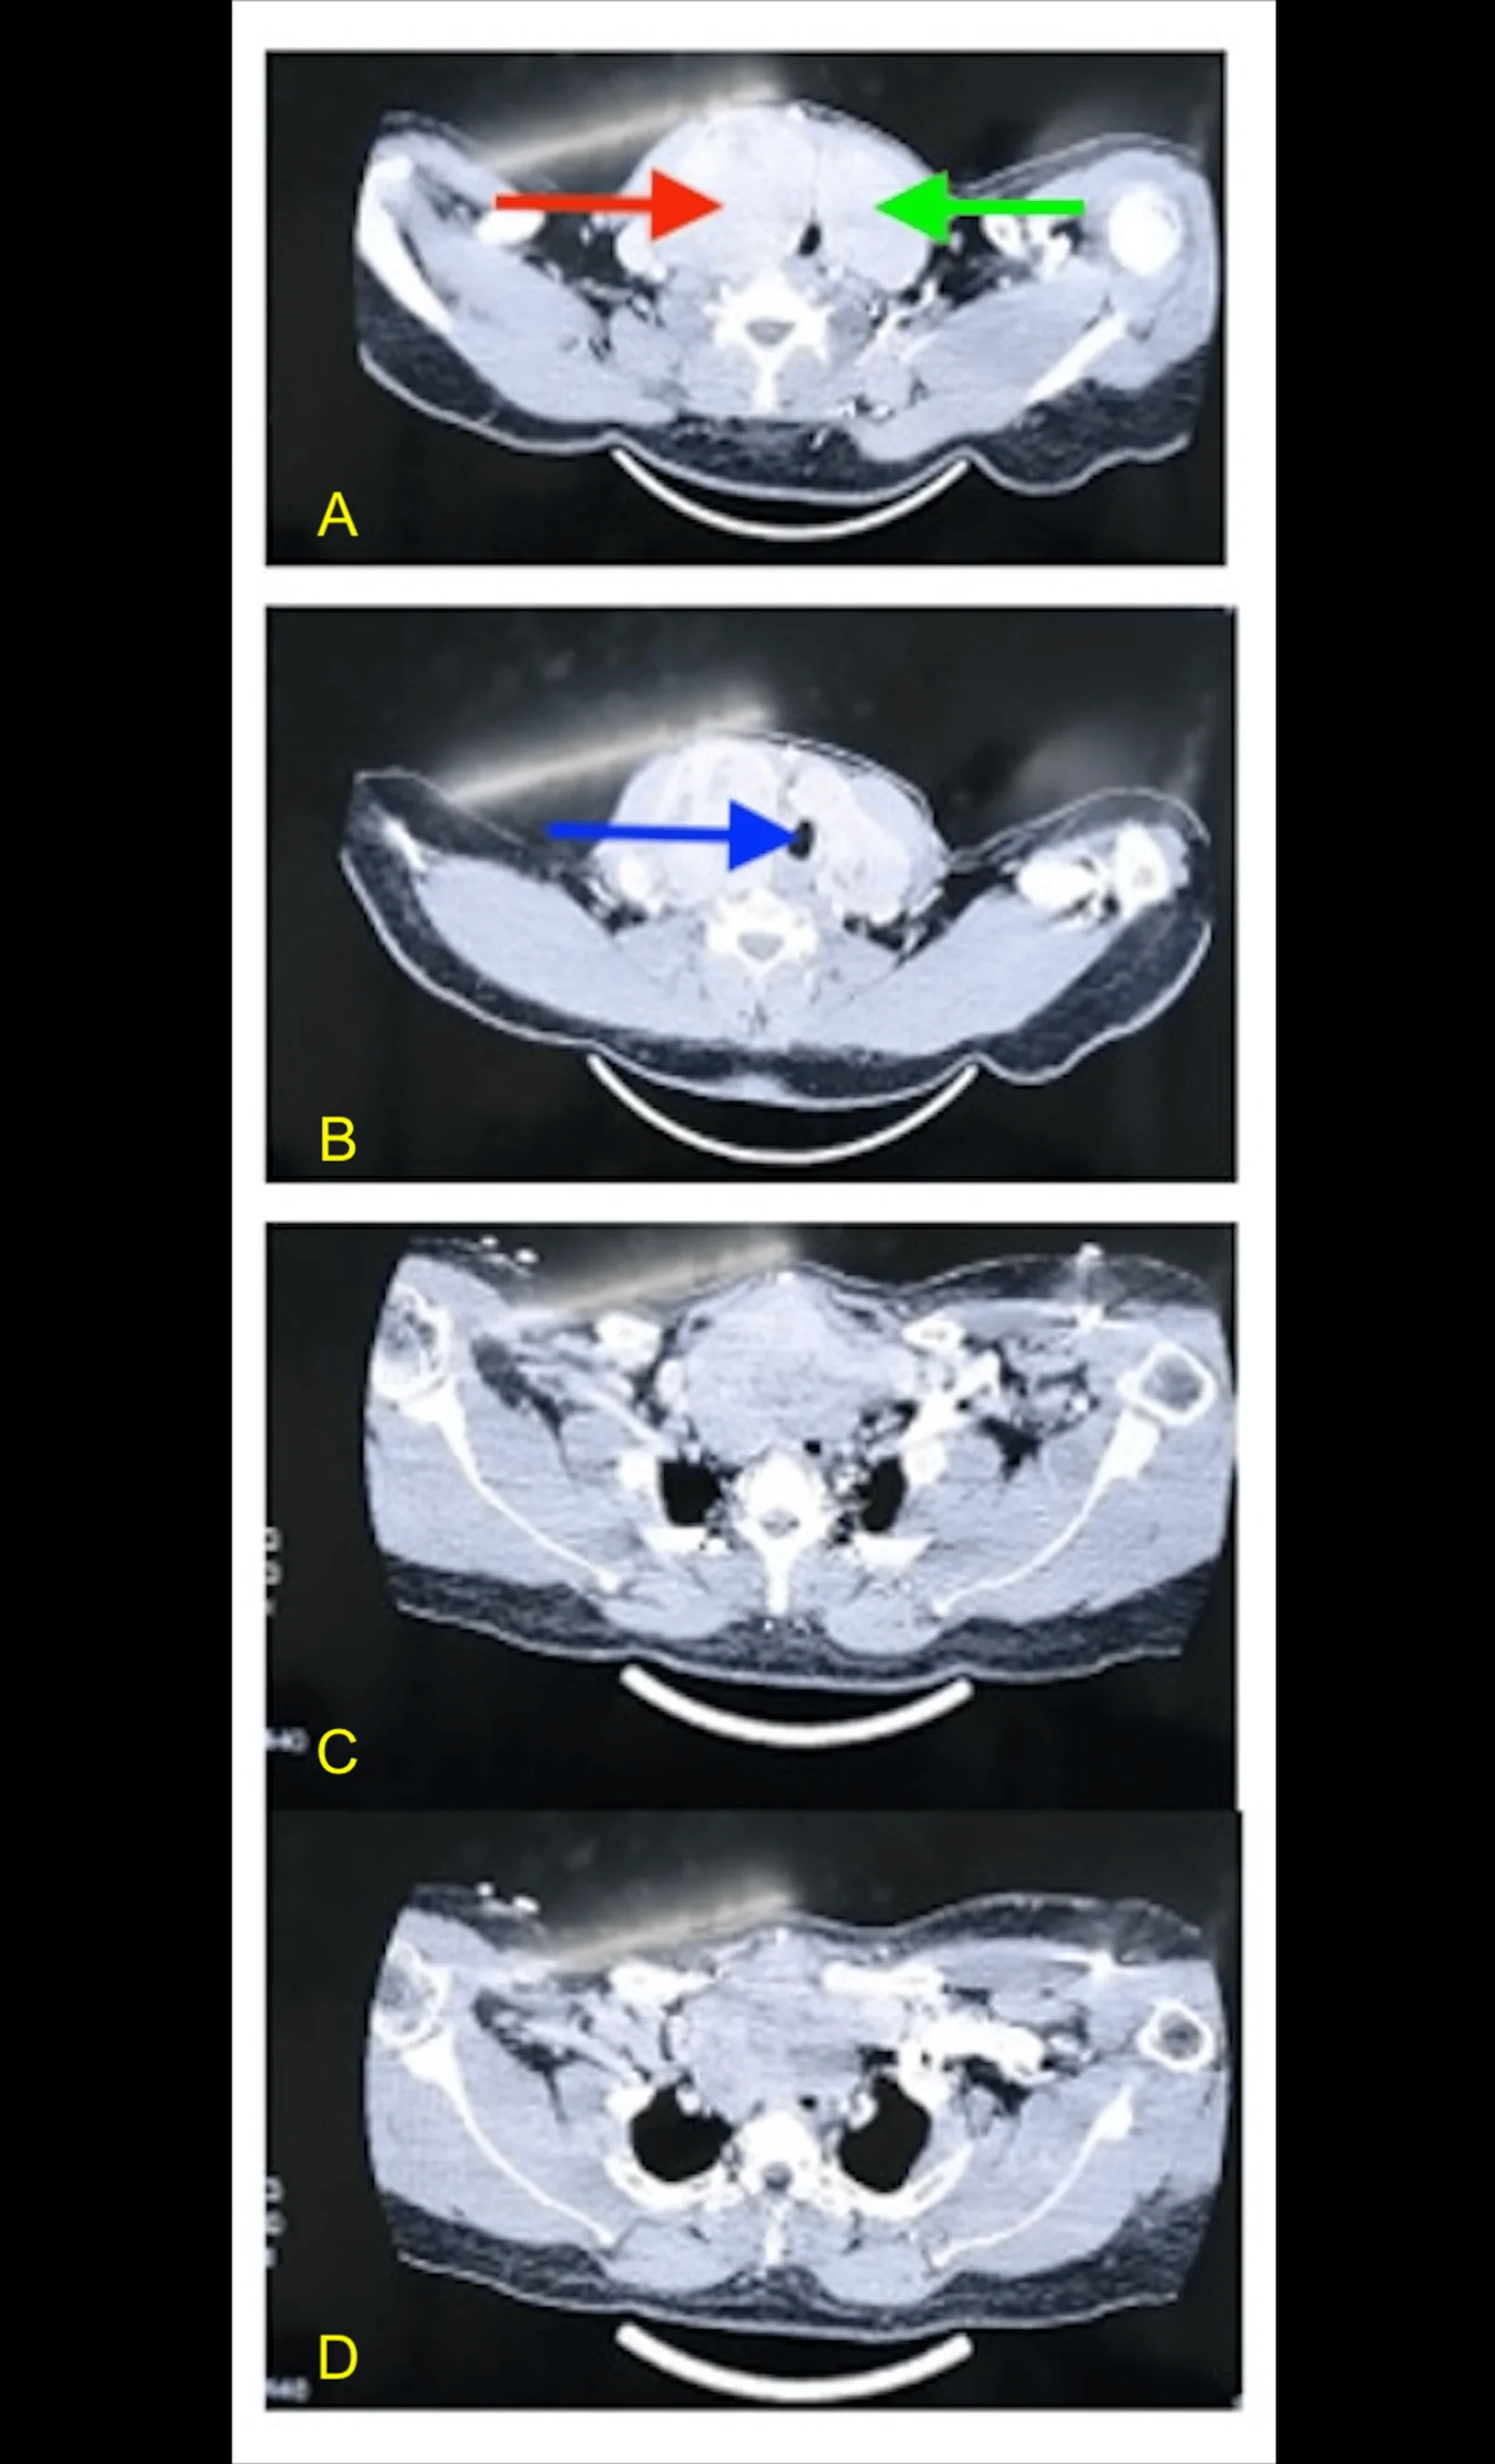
Contrast-enhanced CT demonstrating massive multinodular thyroid enlargement with retrosternal extension and tracheal compression. Panels A, B, C, and D are multiple contiguous images. Red arrow: right thyroid lobe. Green arrow: left thyroid lobe. Blue arrow: trachea.
